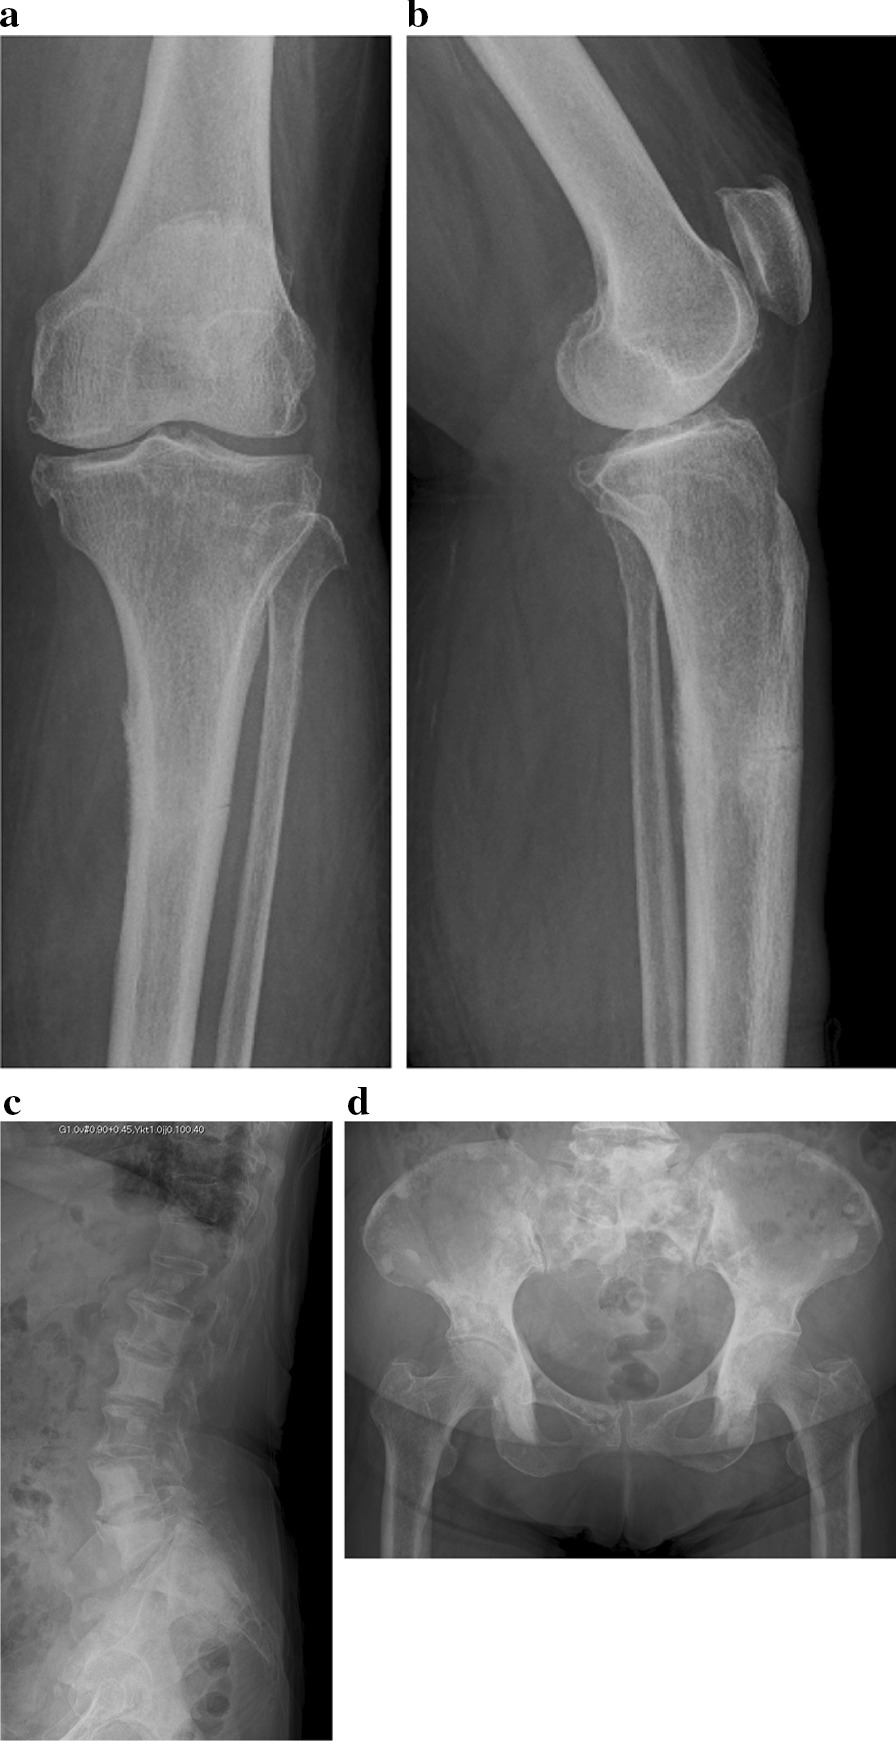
X-ray image demonstrated a non-displaced transverse fracture of the proximal third of the left tibial diaphysis. a Anteroposterior (AP) view of the left tibia. b Lateral view of the left tibia. c Lateral view of the lumber spine. There were multiple metastases of lumber bodies; d anteroposterior X-ray of pelvis. There were also multiple bone metastases
